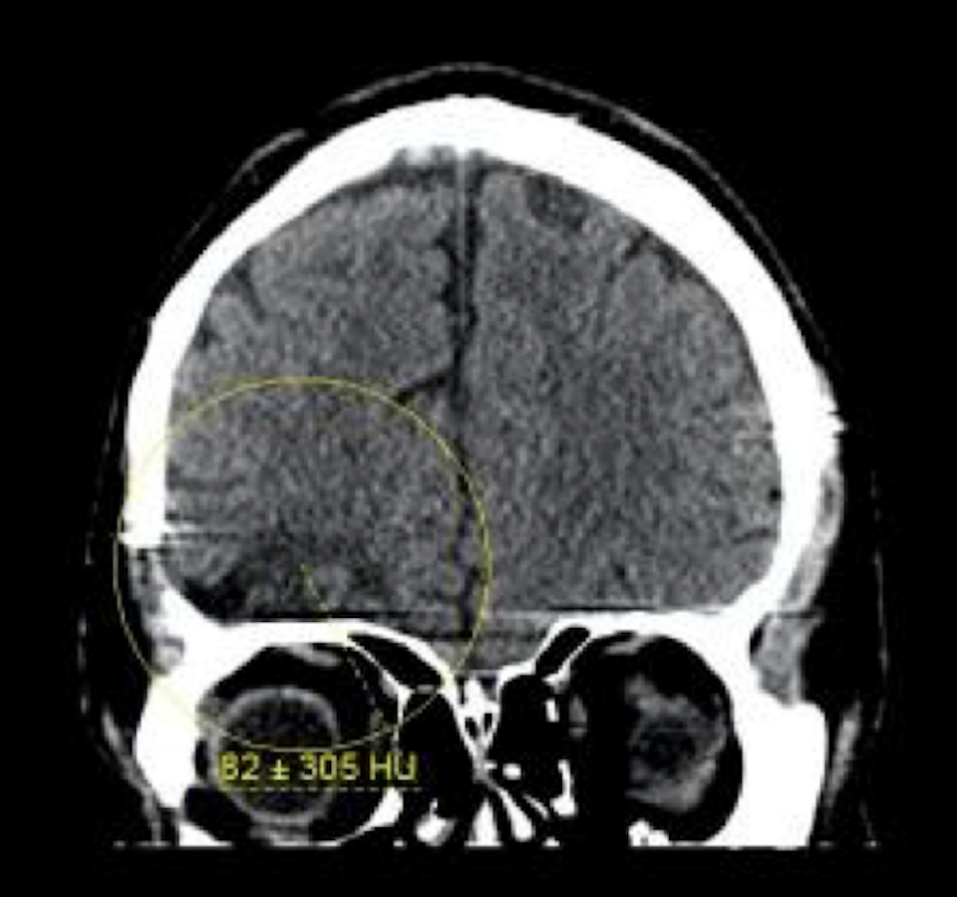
Computed tomography head without contrast axial view. Yellow circle indicates area of encephalomalacia in right temporal lobe.
